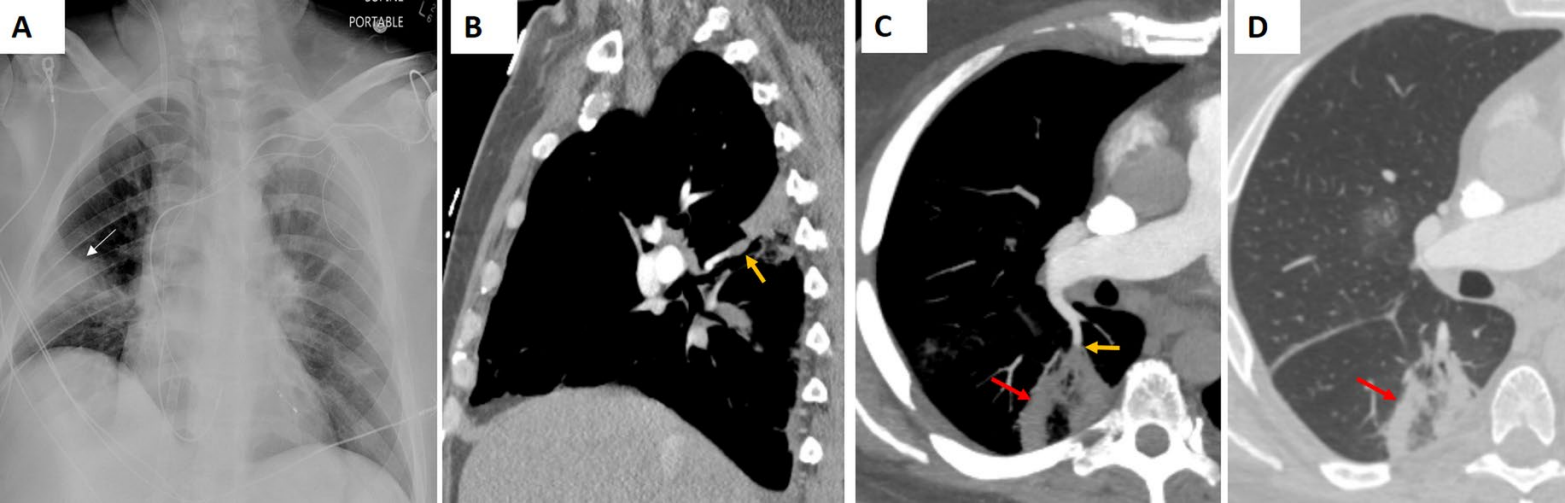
Atoll sign in a patient with pulmonary embolism. The patient had been monitored as an inpatient due to arrhythmia and developed chest pain over the past 24 h. A The frontal radiograph shows a peripheral, wedge-shaped opacity in the right mid-lung (white arrow). B Sagittal and (C, D) axial chest CT angiography images demonstrate pulmonary embolism (orange arrows) and a right lower lobe posterior basilar wedge-shaped pulmonary opacity with the atoll sign (red arrows), consistent with pulmonary infarction
